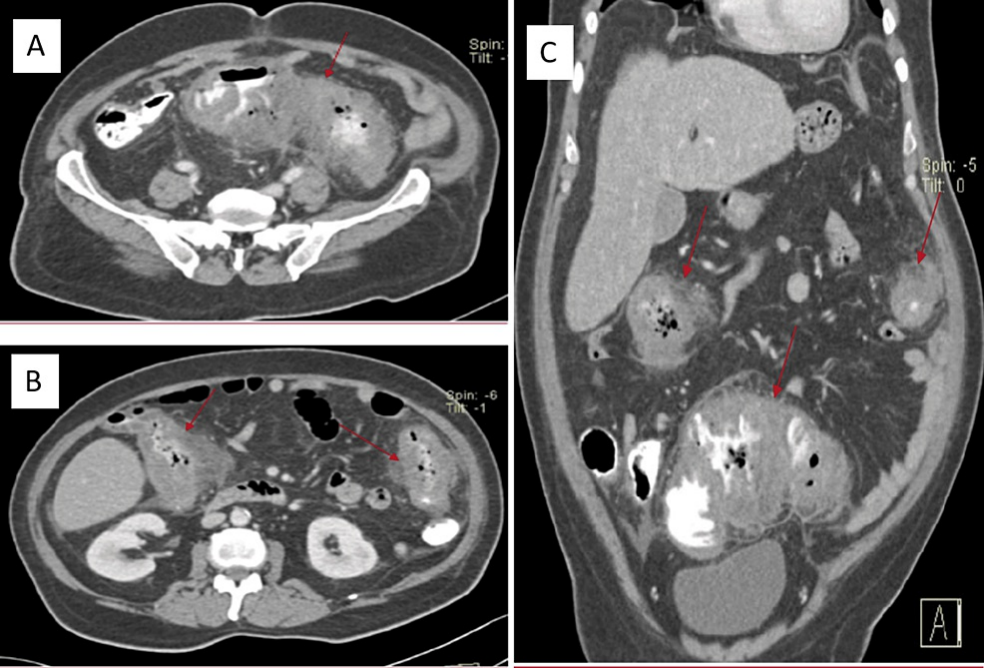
Abdominal contrast-enhanced CT scan (Case 4) Contrast-enhanced CT scan (A, B: axial plane, C: sagittal pane) demonstrates multifocal marked irregular segmental thickening involving the hepatic flexure, splenic flexure, and sigmoid colon, associated with pericolonic inflammatory fat stranding and luminal narrowing (red arrows). No bowel obstruction.
